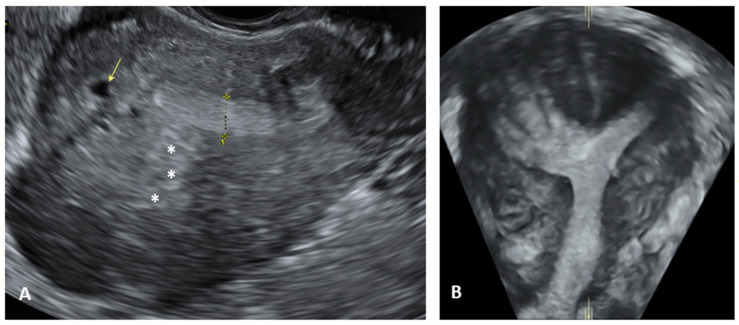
Transvaginal ultrasound (TVS) appearance of adenomyosis. (A) Two-dimensional (2D) TVS evaluation shows direct diagnostic signs: myometrial cystic areas (yellow arrow), hyperechoic foci (with asterisks); (B) Three-dimensional (3D) TVS evaluation of adenomyosis shows a diffuse undefined junctional zone.
